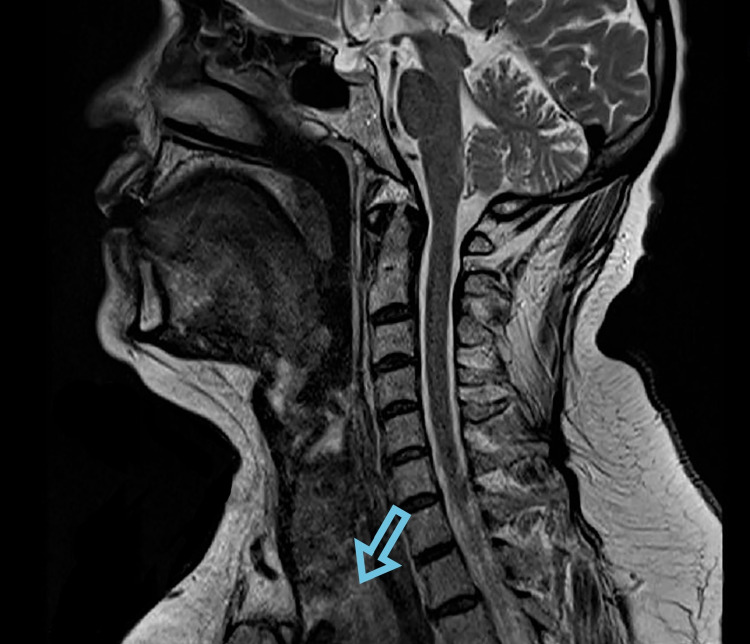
The expansion down of the left inferior lobe of thyroid (arrow), the sagittal section, diagnostic imaging of the neck MRI. MRI: magnetic resonance imaging.
